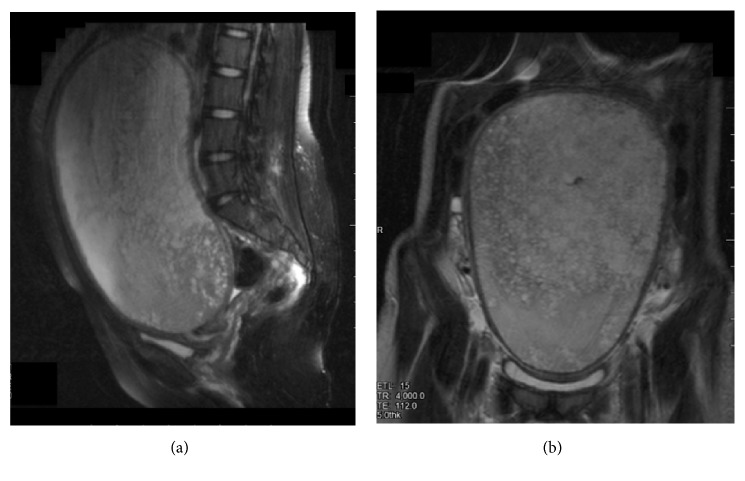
Sagittal (a) and coronal (b) views of an abdominal-pelvic Magnetic Resonance Imaging showing an enlarged uterus (24 × 11 × 17 cm) with a large intrauterine heterogeneous mass.
